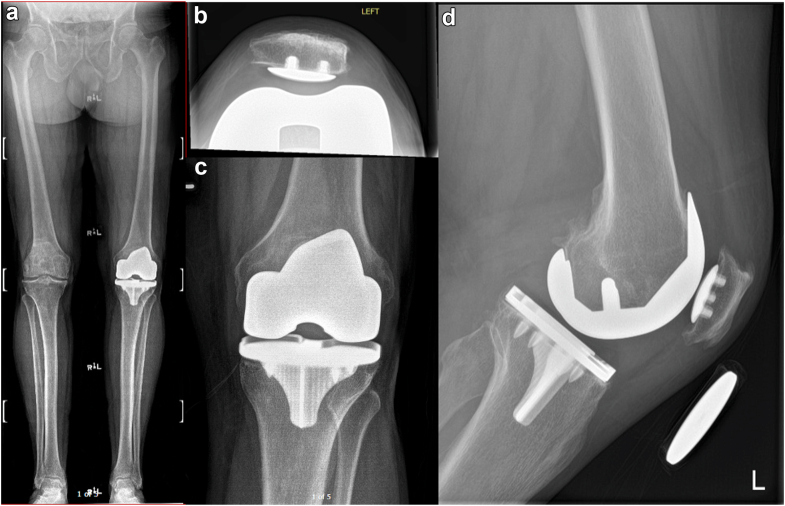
Preoperative radiographs of the left knee prior to rTKA. (a) Long leg standing anteroposterior (AP). (b) Axial patellar view. (c) Knee AP view. (d) Lateral view.
